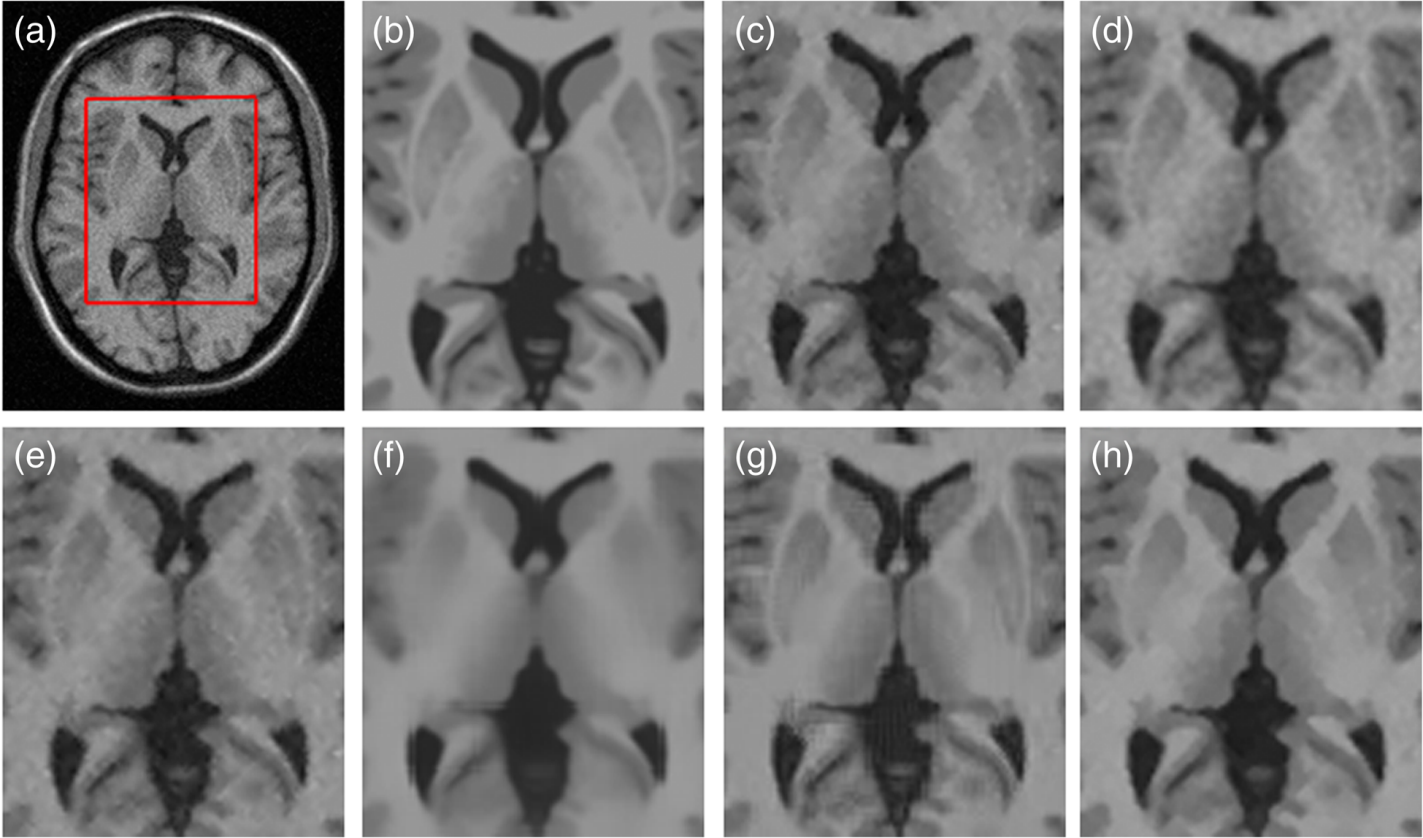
Comparison of visual restoration results on slice 26 with 7% noise in the 3 mm MS dataset. a Noisy MR image. b Intact image in magnified view. c IBF with PSNR = 28.87 and SSIM = 0.7981. d ADF with PSNR = 31.41 and SSIM = 0.8133. e LMMSE with PSNR = 29.08 and SSIM = 0.8016. f NLM with PSNR = 31.74 and SSIM = 0.8406. g BM3D with PSNR = 31.78 and SSIM = 0.8367. h Proposed with PSNR = 31.95 and SSIM = 0.8479
